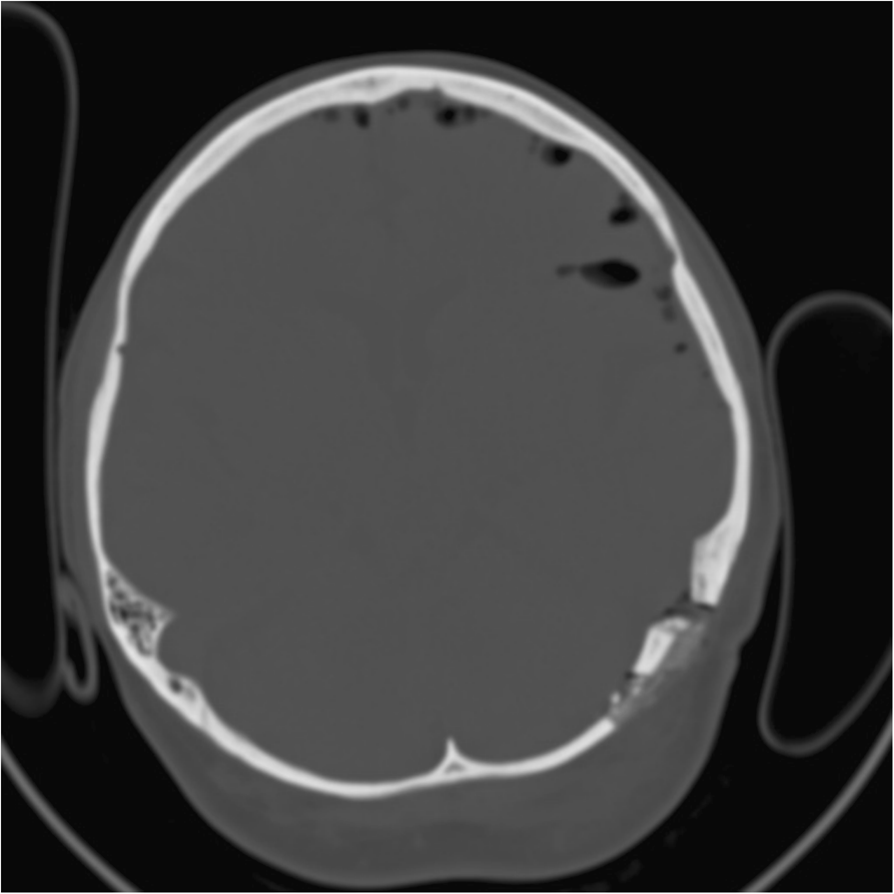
Axial bone-window computed tomography scan performed one day after first operation. The mass of bone chips and fibrin glue can be well identified.
